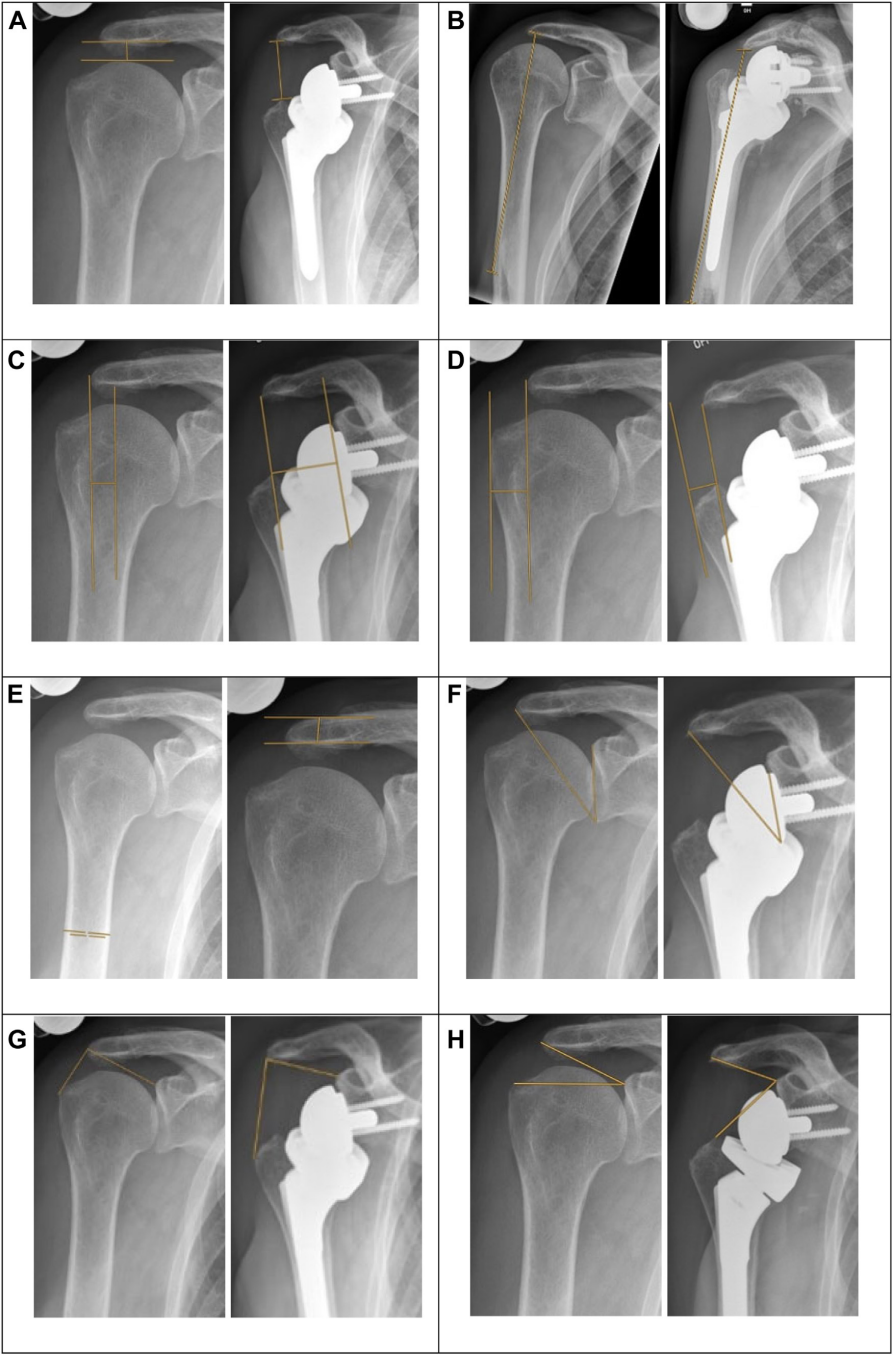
The figure visualizes the measurement of predictive preoperative and postoperative radiographic parameters on an a.p. view. The method is shown as *yellow line* for (A) acromiohumeral distance (ACHD), (B) deltoid length, (C) distance from center of rotation to the lateral acromion (COR-LA), (D) distance from the lateral acromion to the most lateral tip of the greater tuberosity (LA-GT), (E) deltoid tuberosity index (DTI) and acromial thickness, (F) critical shoulder angle (CSA), (G) lateralization shoulder angle (LSA), and (H) distalization shoulder angle (DSA). The measurement was explained previously.^16^. Reprinted with permission from Copyright Clearance Center Inc. on behalf of the rightsholder Elsevier Science & Technology Journals (ID 1294307-1).^16^
